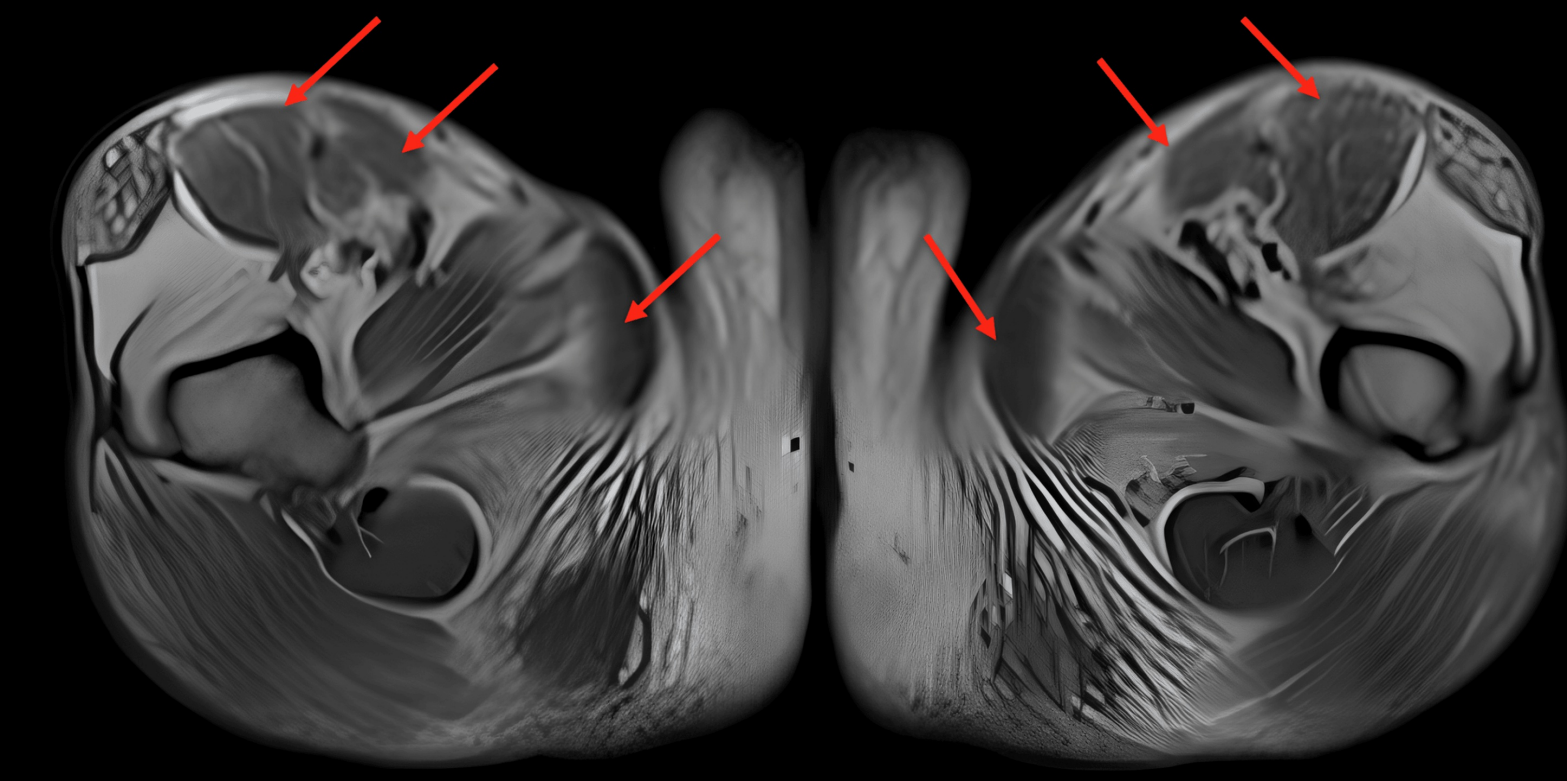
MRI bilateral thigh. MRI showed fatty infiltration in bilateral thighs with relative sparing of bilateral gracilis, sartorius, and rectus femoris muscles (medial to lateral arrows) in each side.
